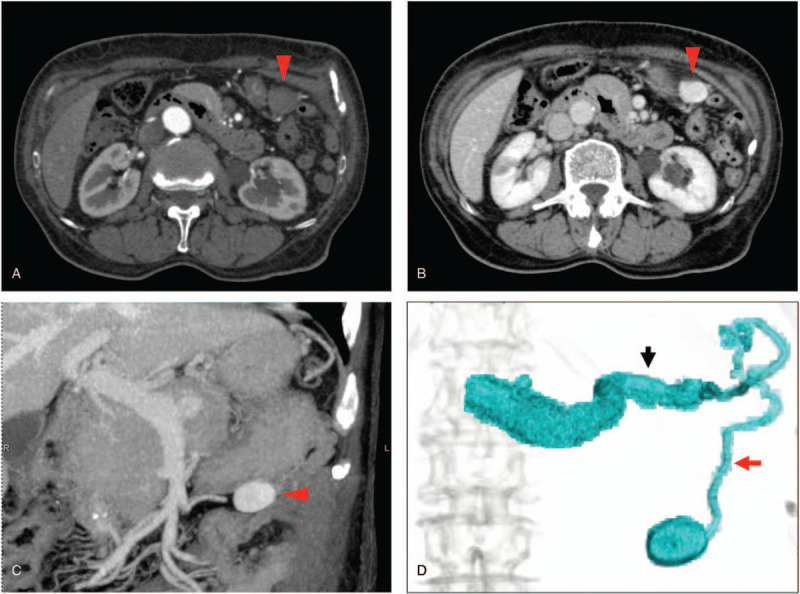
Representative preoperative images. (A) An axial contrast-enhanced CT image in the early phase demonstrating that the tumor of the abdominal cavity was not enhanced heterogeneously (red arrowhead). (B) The same area presented in the late phase, showing enhancement (red arrowhead). (C) A coronal enhanced-CT image in the late phase. These images demonstrate that the enhanced tumor was situated at the greater curvature (red arrowhead). (D) 3D-CTA. These images demonstrate that the tumor was supplied from the LGV (red arrow) and the splenic vein (black arrow).
